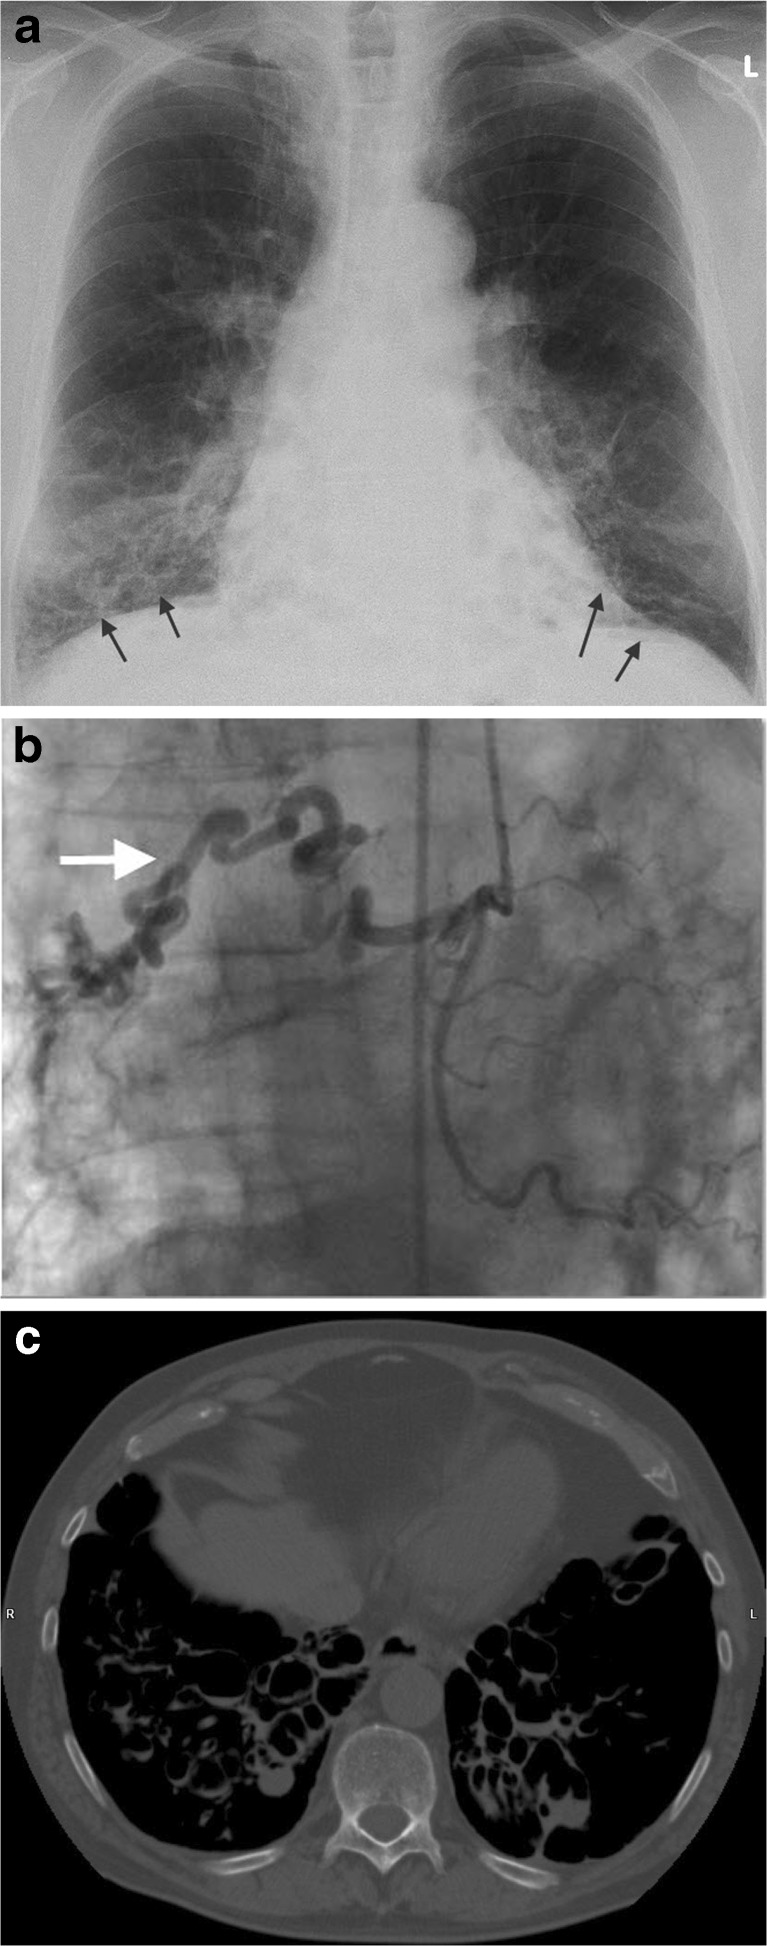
a Chest X-ray demonstrating bronchiectasis at the right and left lower regions of the lung (*arrows*), b Coronary angiographic frame of RCA in AP position, depicting the CBF between a proximal branch of the RCA and bronchial artery (*arrow*) and c Thoracic computed tomography scan showing the bilateral bronchiectasisConventional CAG demonstrated one-vessel disease with a subtotal stenosis in the proximal LAD and fistulous multiple tiny vessels exiting from a proximal branch of the small calibre RCA with a possible connection to the right pulmonary artery and right bronchial artery (Fig. 2b). At cardiac catheterisation mild pulmonary hypertension (capillary wedge pressure 16, RV pressure 53/9 and pulmonary artery pressure 40/16 mmHg), normal cardiac output (6.0 ml/min) and on oximetric series no left-to-right shunt was found. MDCT confirmed bilateral cystic bronchiectasis of the basal pulmonary fields and left middle lobe (Fig. 2c). The LAD showed calcification with suspected high-grade stenosis, a normal Cx and RCA. Furthermore, a CBF from the proximal part of the RCA communicated with the right bronchial artery. Radionuclide shunt measurement and MPI demonstrated a fixed defect in the anterior and apical region without reversibility with an LV ejection fraction of 48 % without a detectable left-right shunt. Pulmonary ventilation/perfusion scintigraphy revealed bilateral matched wedge-shaped lesions in the basal lung segments excluding pulmonary embolism. Cardiovascular magnetic resonance investigation (CMR) showed bilateral pulmonary lesions and no viable myocardium of the anterior wall with moderate LV dysfunction. PCI was abandoned and medical management was instituted. He was treated with metoprolol, acetylsalicylic acid, perindopril, simvastatin, tiotropium, acetylcysteine, and formoterol/beclometasone. He remains free of symptoms and was scheduled for annual follow-up.
